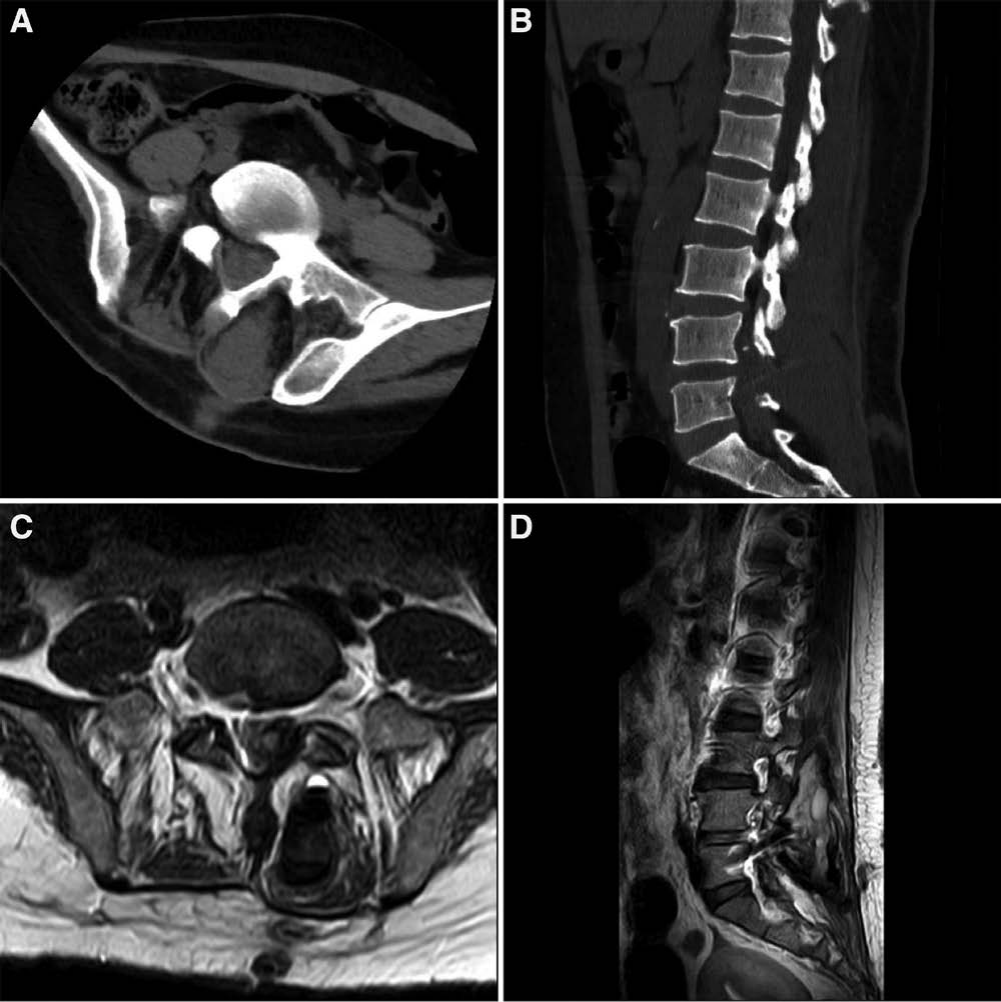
CT (A, B) and MRI (C, D) of the lumbar vertebrae showed the possibility of multiple hematomas within the vertebral canal and in the left posterior aspect of the vertebral body and subcutaneous adipose tissue.
